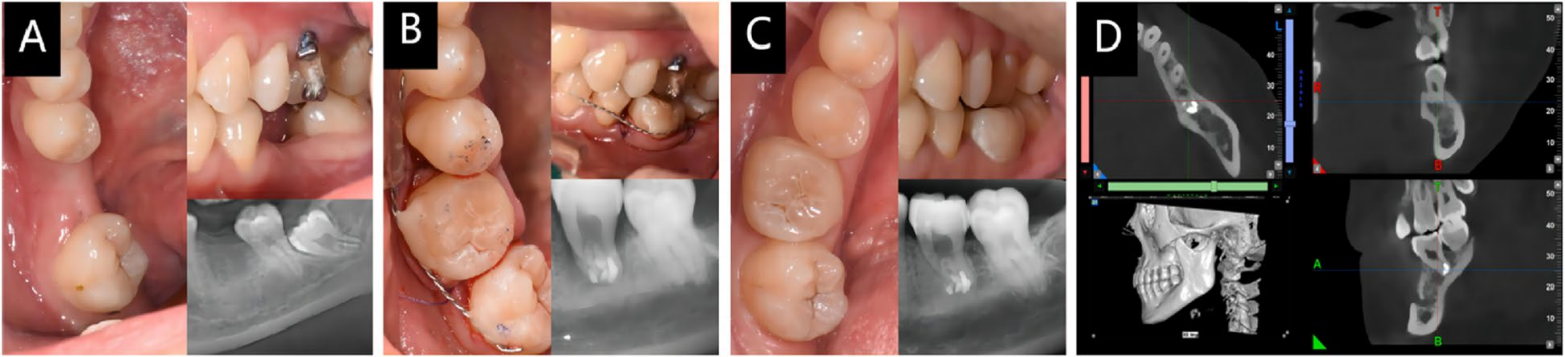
Preoperative clinical photographs and PA of the recipient area (A); clinical photos and PA of the recipient area immediately after surgery (B); clinical photos, PA and CBCT of the recipient area at 28 months after surgery (C, D)
